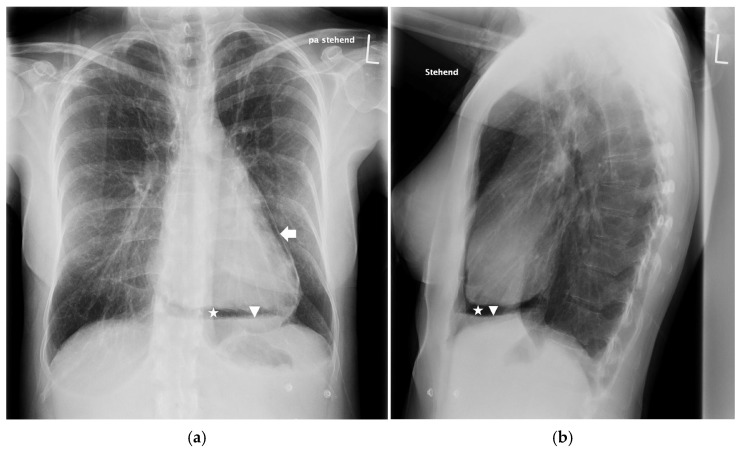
Posterior–anterior (a) and lateral (b) chest X-ray showing a radiolucent rim (star) separating the pericardium (arrow) from the cardiac silhouette with an air–fluid level (arrow head) within the pericardial space.
